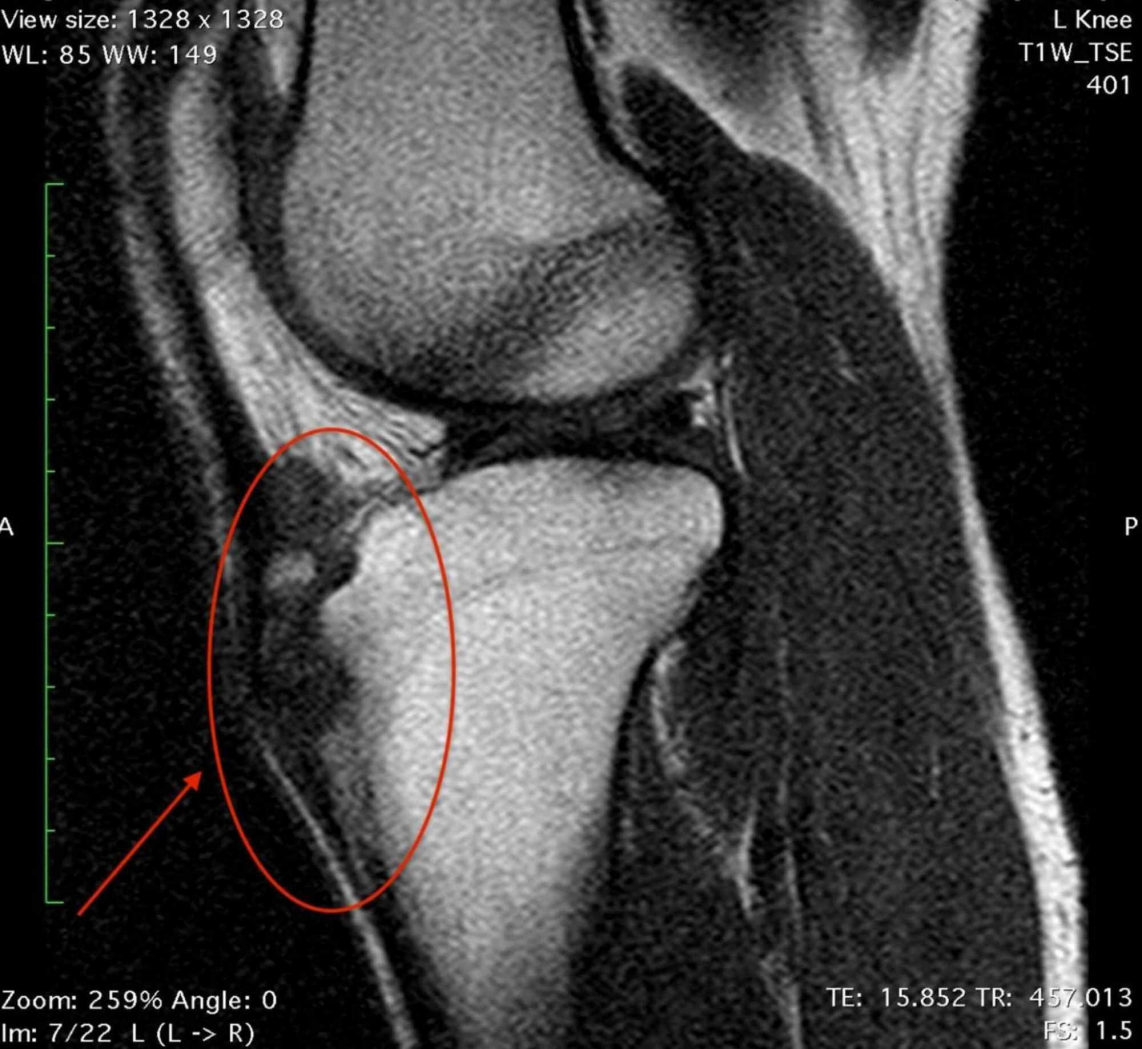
MRI scan showing the ossicle.
